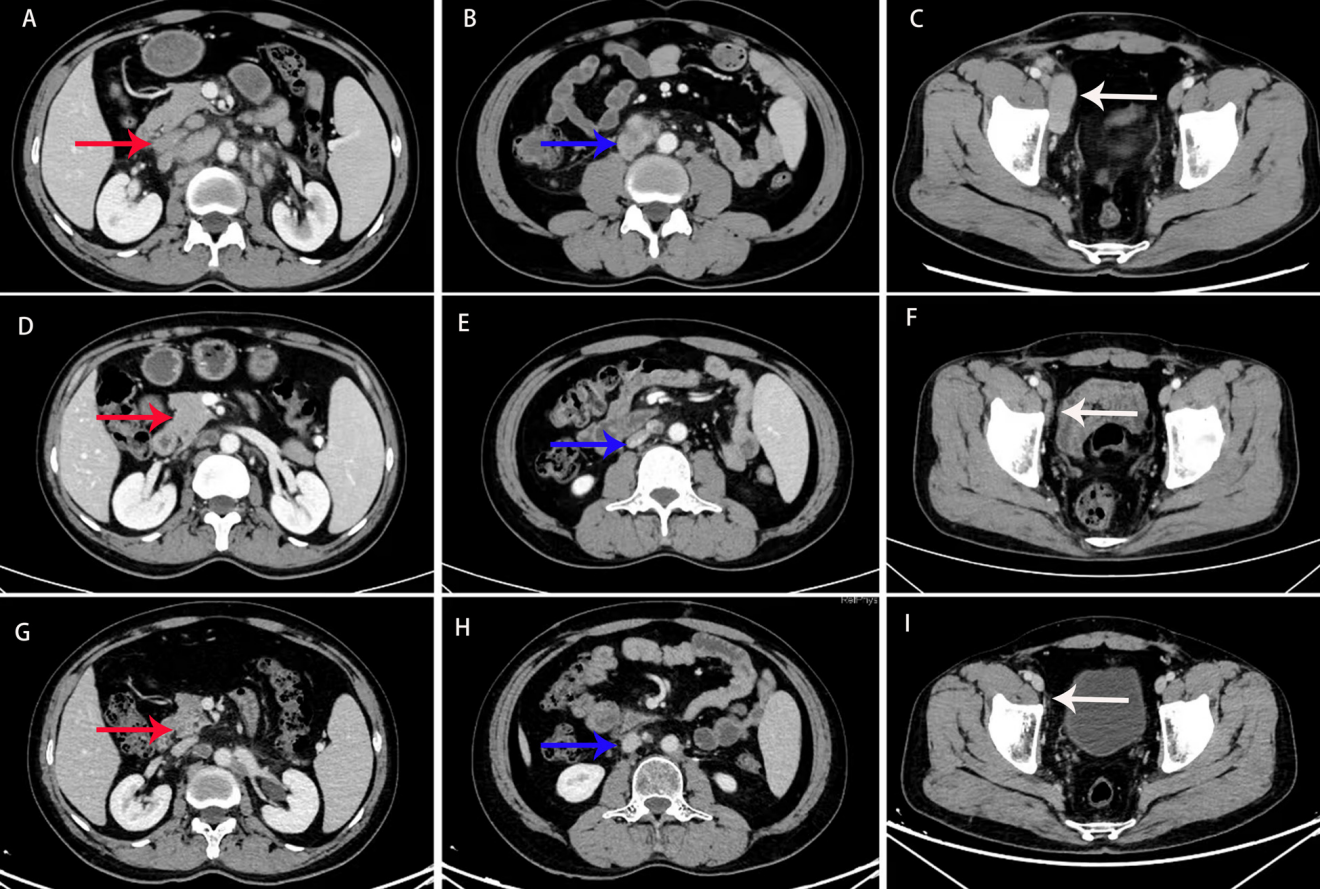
Computed tomography (CT) scan before treatment (A–C), and 6 (D–F) and 30 months (G–I) after treatment. CT imaging revealed extensive metastasis in the retroperitoneal and pelvic lymph nodes (indicated by the red, blue, and white arrows). After 6 and 30 months of treatment, the lesions showed a significant reduction, and the therapeutic response was evaluated as a partial response (PR).
